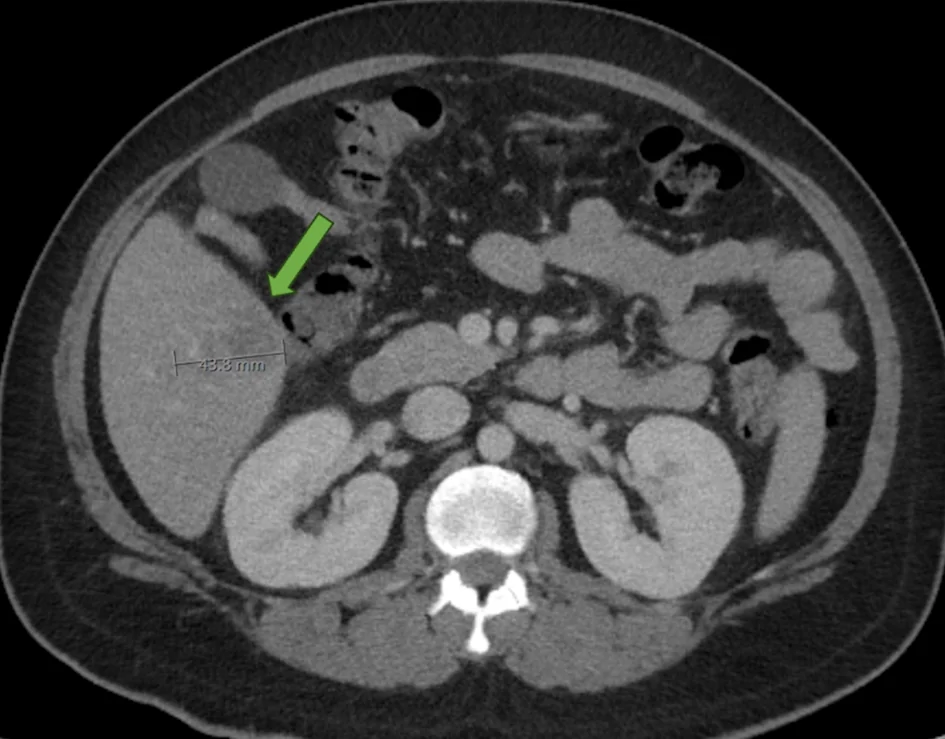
Contrast-enhanced axial abdominal CT image of the abdomen showing a 43.8-mm ill-defined hypoattenuating lesion in the inferior right hepatic lobe. The arrow identifies the larger hepatic lesion. CT: computed tomography.
